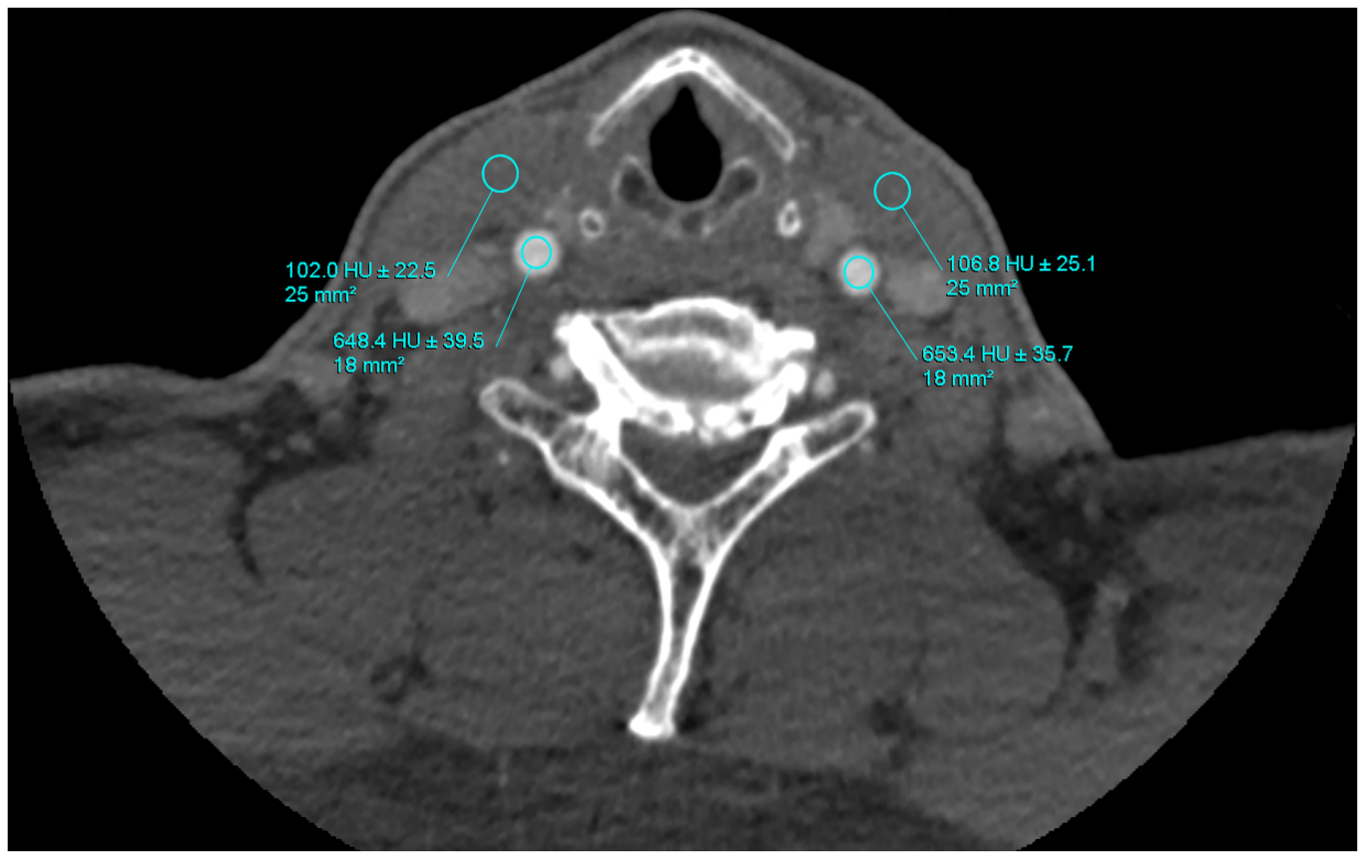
Determination of image noise, vascular attenuation. The CT attenuation value was measured at circular regions of interest (ROI) placed in the center of common carotid arteries. Data were shown as mean ± standard deviation. Additional ROIs were placed at the sternocleidomastoid muscle adjacent to the vessel contour.
